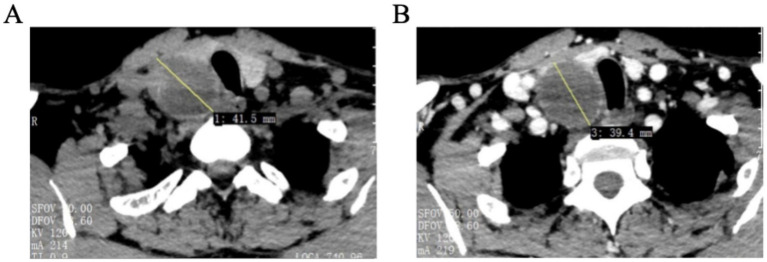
(A) Plain scan CT and (B) enhanced CT. Cervical plain plus contrast-enhanced CT findings were: 1. Hypodense nodular shadow of the left thyroid gland; 2. Oval cystic solid mass shadow of the right inferior thyroid gland, and parathyroid adenoma with partial cystic degeneration after first examination (please combine with other related examinations).
